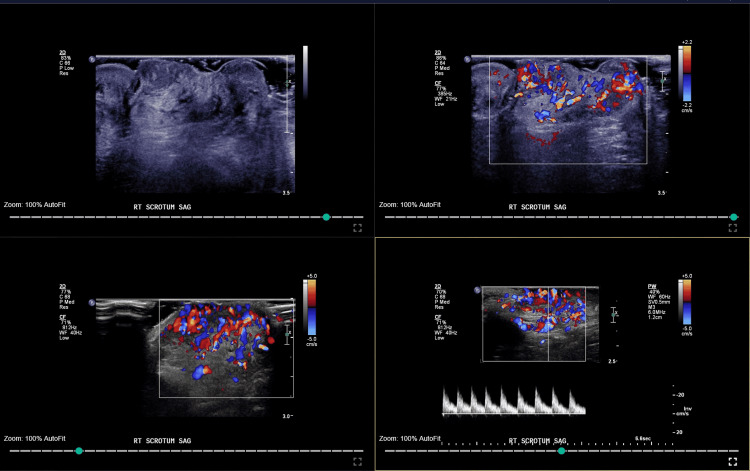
Doppler ultrasonography scan of the scrotum showing the heterogeneous hypervascular lesion
